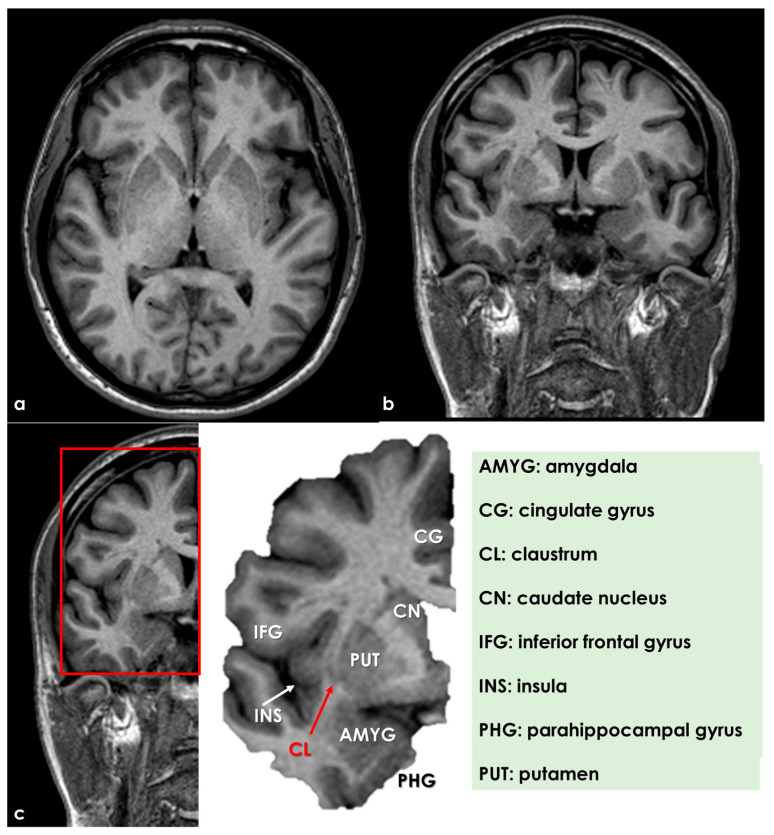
Brain magnetic resonance imaging (MRI) showing the localization and neighbor structures of the human claustrum. Panel (a,b) show axial and coronal T1W MRI, respectively, at the level of the insula in a normal subject. Panel (c) shows a detail of the right hemisphere in the coronal plane and a further magnification of the brain structures behind the claustrum (right portion of the panel).
